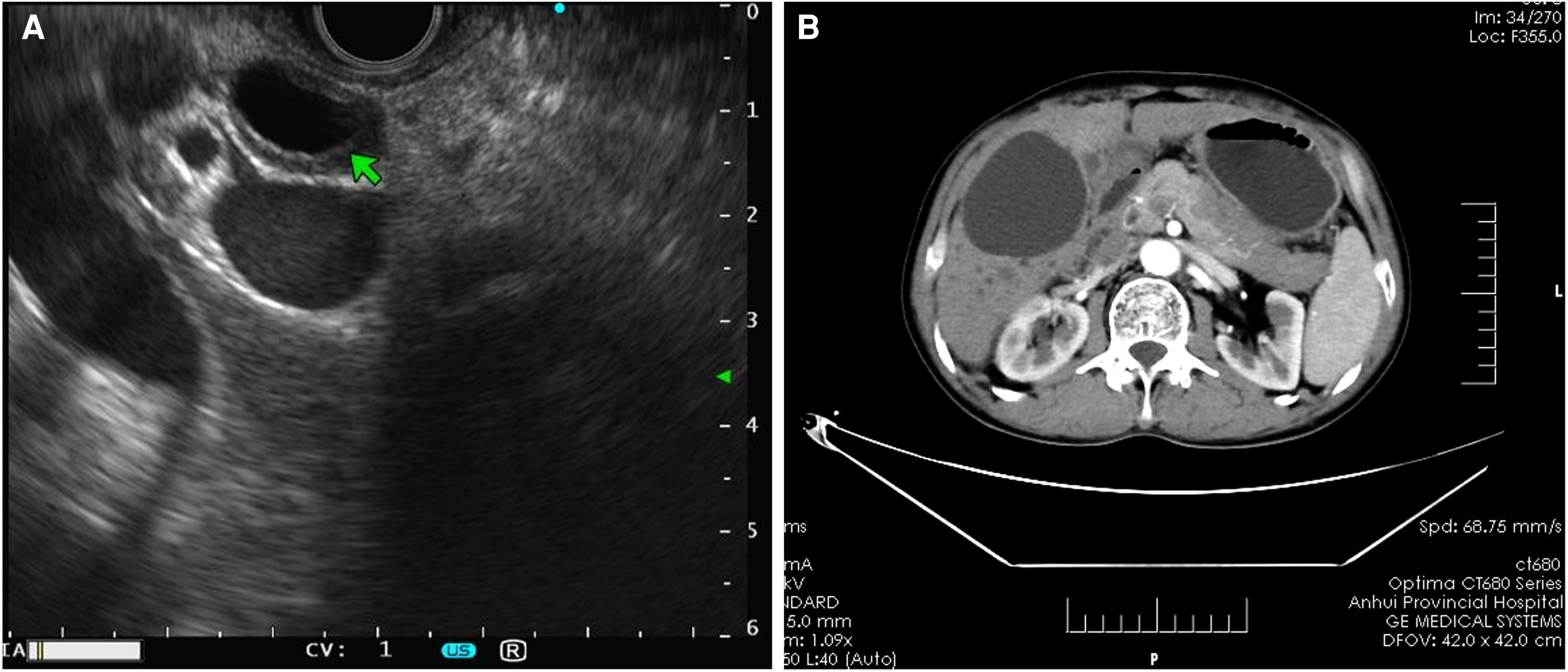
Imaging features of AIP. (A) Endoscopic ultrasound revealed bile duct wall thickening and common bile duct dilatation with the bilateral sign in patients with AIP. (B) Computed tomography scan showed an even thickening of the common bile duct wall, evident enhancement in the arterial phase, and uneven and irregular enhancement of the head and neck areas. AIP, autoimmune pancreatitis.
